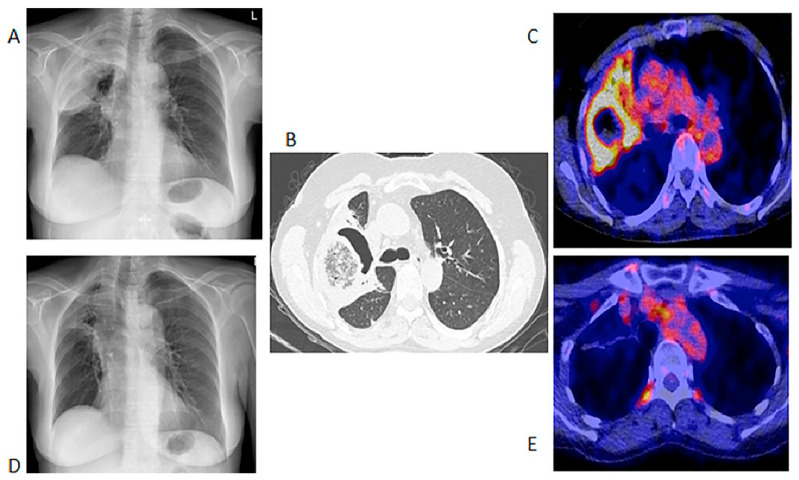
Chest X-ray, HR CT and [^18^F]FDG PET/CT of a 60-year-old female with acute myeloid leukemia on chemotherapy. She had a proven fungal infection (*Alternaria alternata*). (A)—Initial chest X-ray. (B)—Initial HR CT scan performed before the [^18^F]FDG PET/CT. (C)—Fused axial image of [^18^F]FDG PET/CT of the chest. After 2 months of antifungal therapy. (D)—Chest X-ray showing response with a residual lesion. (E)—Fused axial [^18^F]FDG PET/CT showing an almost complete metabolic response in the IFI lesion. The data provided by [^18^F]FDG PET/CT at follow-up were deemed adequate, so patient did not require a repeat HR CT.
